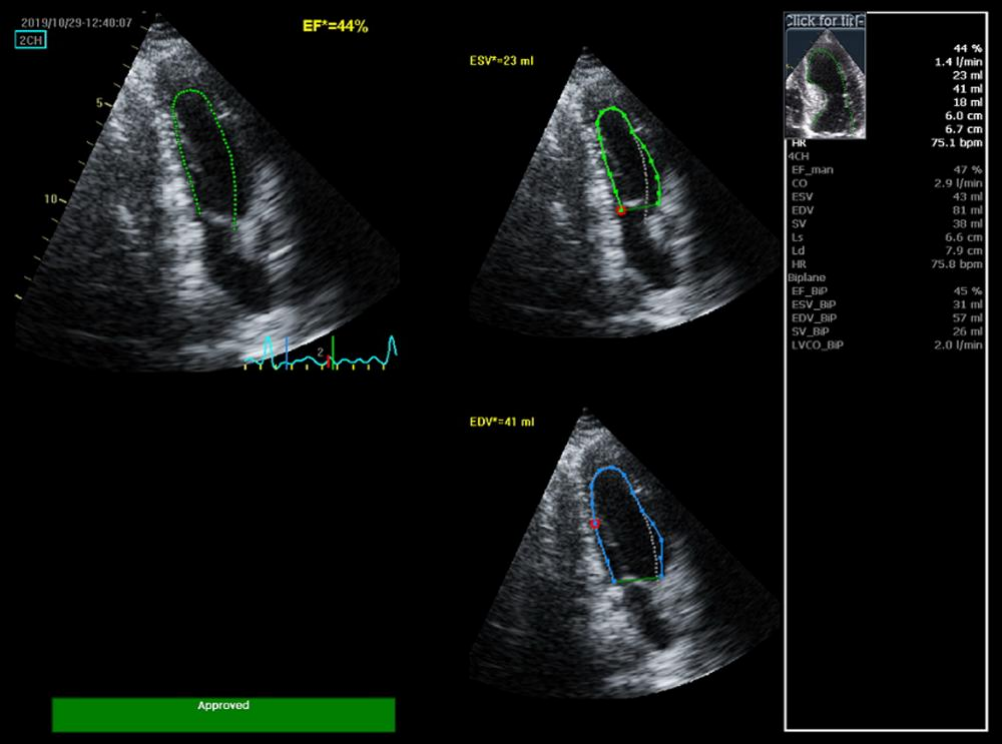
Initial transthoracic echocardiogram showing moderately impaired left ventricular (LV) systolic function, with Simpson's biplane ejection fraction of 44%.
